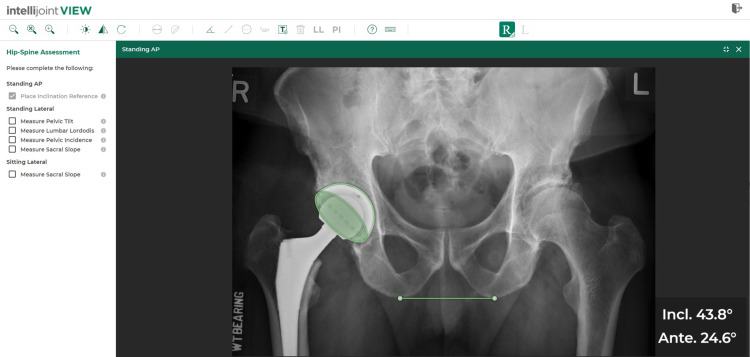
Measurement using Intellijoint VIEW. Sample AP pelvic radiograph with acetabular component position and leg length measurements, as measured using Intellijoint VIEW. AP: anteroposterior
